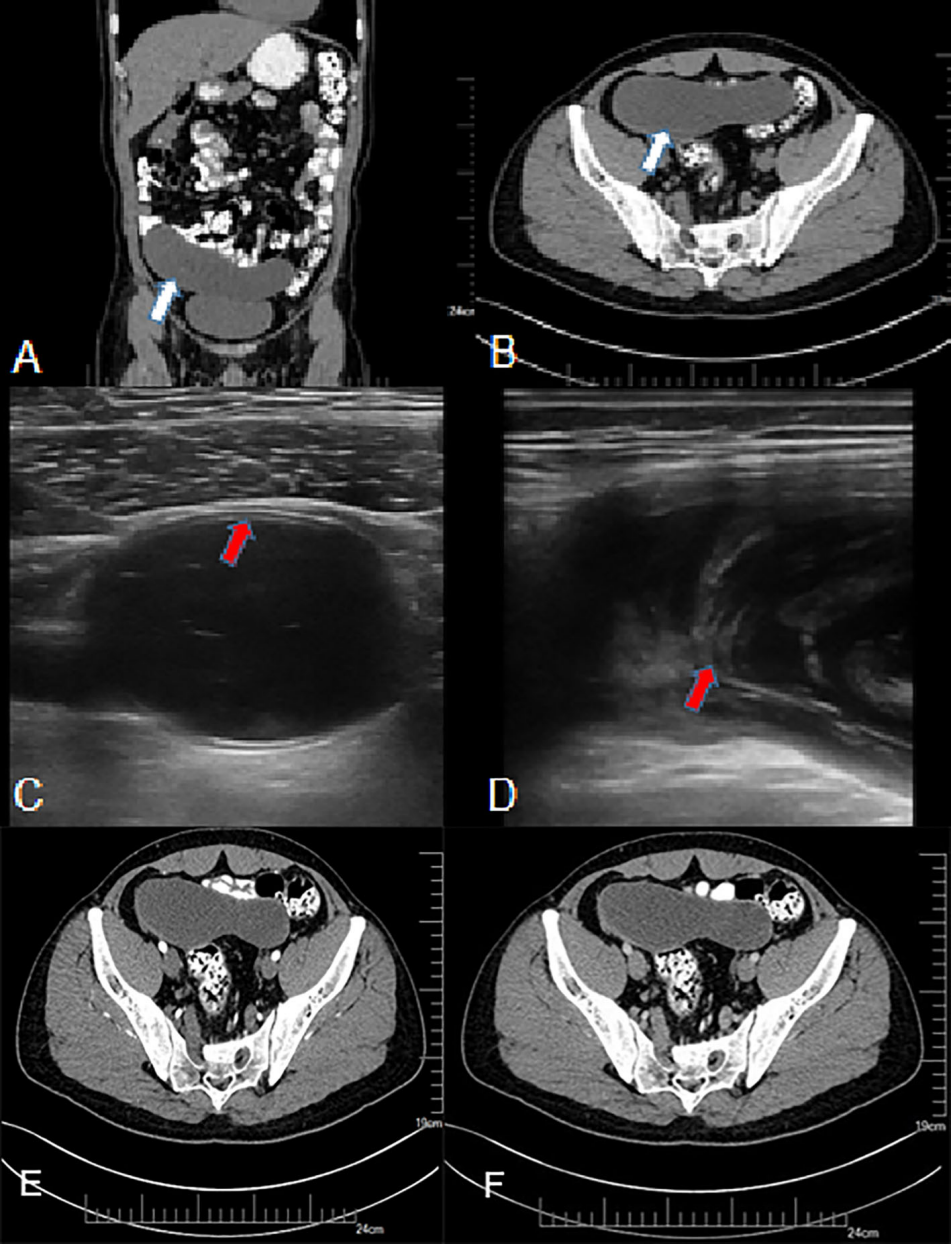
A 50-year-old man with LAMN. (A, B) Plain CT scan, showing significant widening of the appendix, a thin-walled low-density focus (white arrow), with uniform density and clear surrounding fat space. (C, D) US examination, (C) showing clear layer of the appendix wall (red arrow) and (D) showing flocculate echo in the appendix cavity (red arrow). (E, F) CT enhancement; after enhancement, there was no clear enhancement in the center of the lesion, and mild delayed enhancement was observed at the edge.
